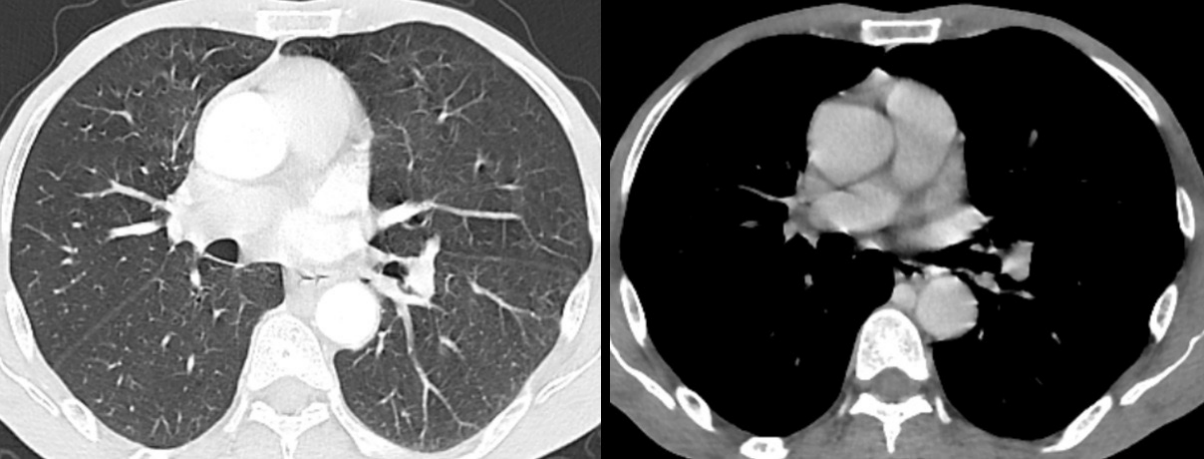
Axial CT scans of the chest in lung parenchyma and mediastinal window showing no lung lesion and no mediastinal lymphadenopathy.
